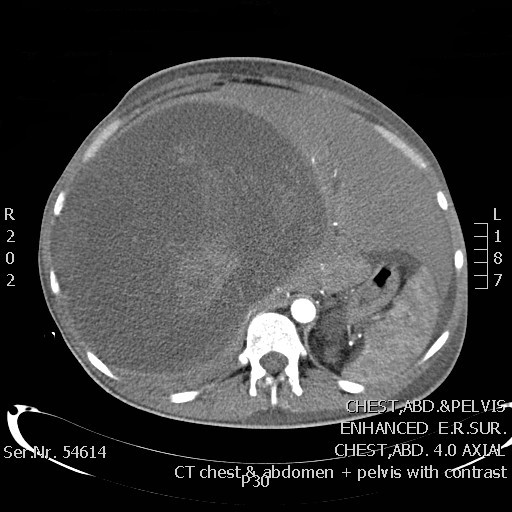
CT scan of patient number 3 with primary hepatic sarcoma.
